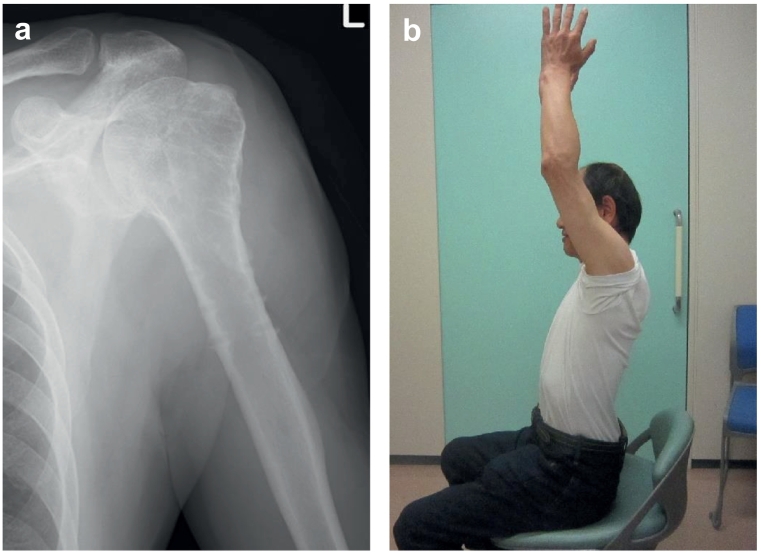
a: Postoperative shoulder radiograph after screw and plate removal b: Shoulder elevation by the patient 3 months after screw removal.
